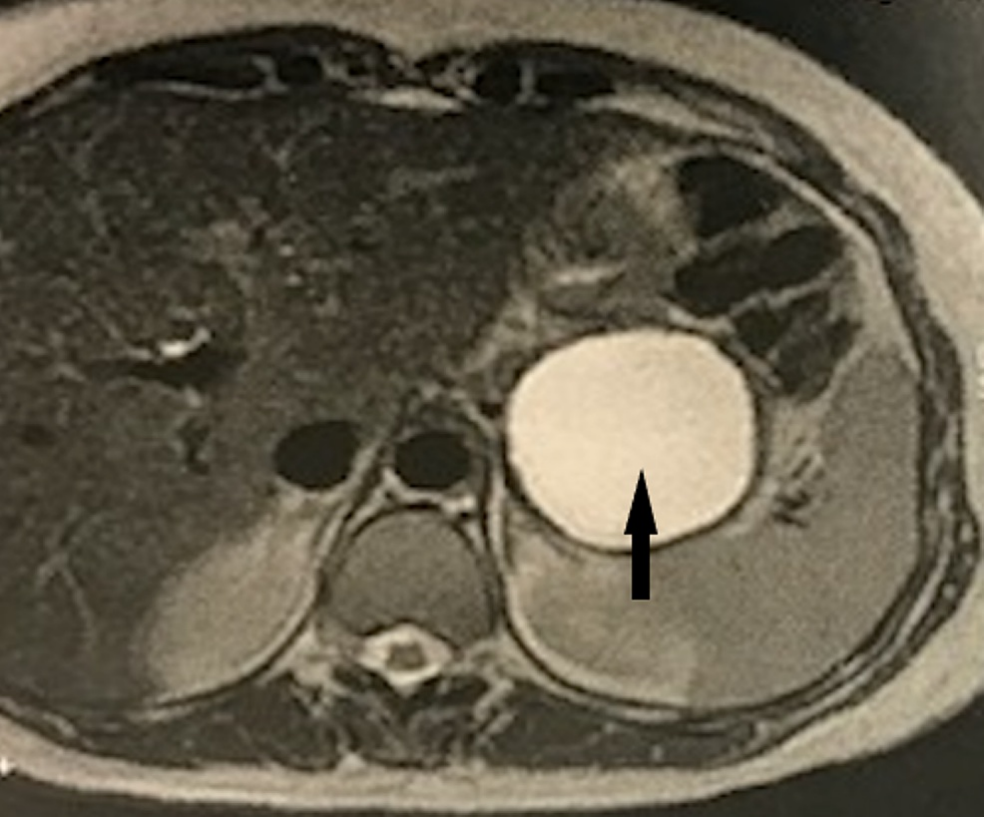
A pancreatic MRI suggesting a mucinous cystadenoma of the tail of the pancreas. MRI: magnetic resonance imaging
